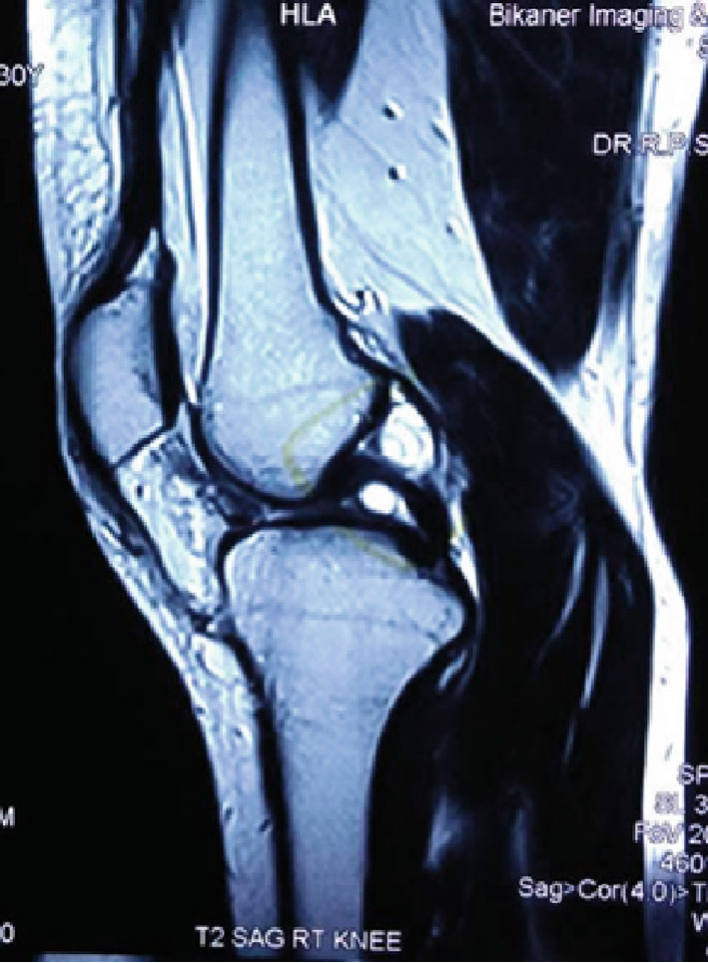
Saggital section of MRI showing cyst between the femoral attachment of two cruciate ligaments.
